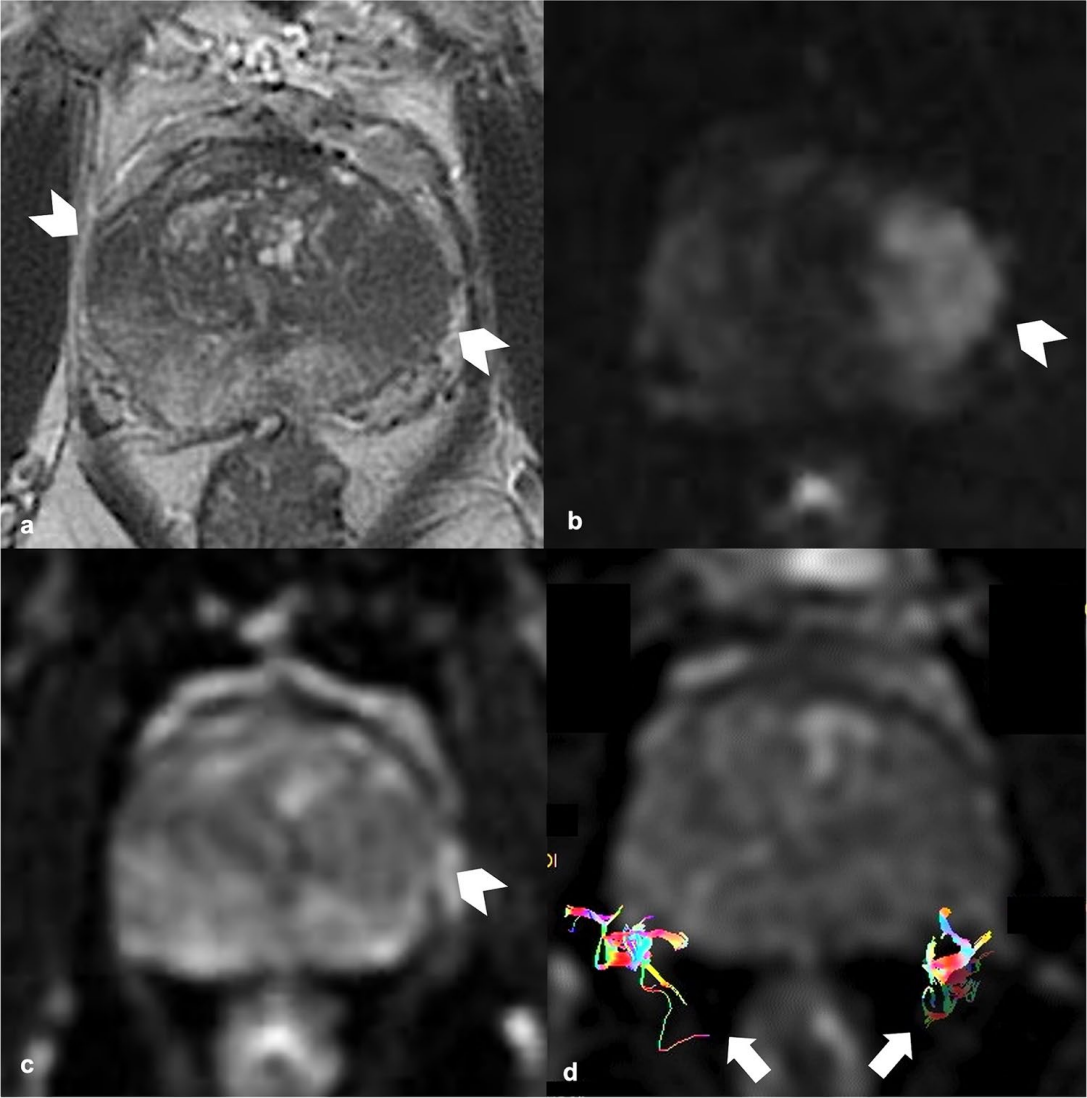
a T2WI (axial) shows two alterations respectively on the left lateral mid peripheral zone (white arrow head), which determines capsular bulging and on the right mid antero-lateral peripheral zone (white arrow head); b, c DWI (b = 2000) and ADC map show a focal and marked hyperintensity on high b-value and focal hypointensity on ADC map on the left lateral peripheral zone lesion (white arrow head), with capsular bulging. d DTI reveals that the lesion is distant from the NVB (white arrows). T2WI, T2 weighted imaging; DWI, Diffusion Weighted Imaging; ADC map, Apparent Diffusion Coefficient map; DTI, Diffusion Tensor Imaging; NVB, neurovascular bundle
